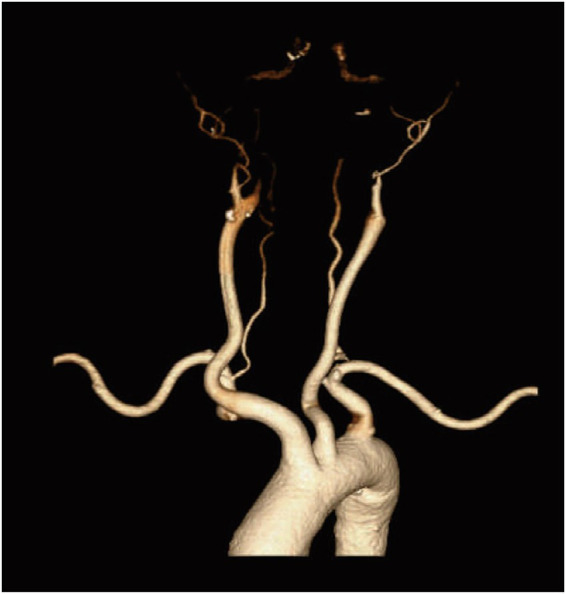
The head CT angiography (CTA) examination reveals non-opacification of bilateral distal carotid arteries, indicating intracranial hypertension.
